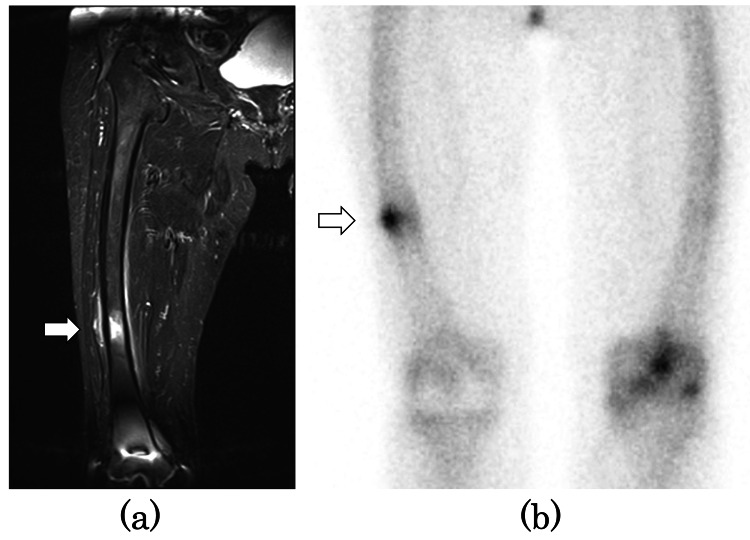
Preoperative MRI STIR coronal image and bone scintigraphy (a) MRI STIR coronal image showed bone marrow edema in the diaphysis of the right femur (white arrow). (b) Bone scintigraphy showed a stress fracture of the right femur (white arrow). MRI: magnetic resonance imaging, STIR: short tau inversion recovery
